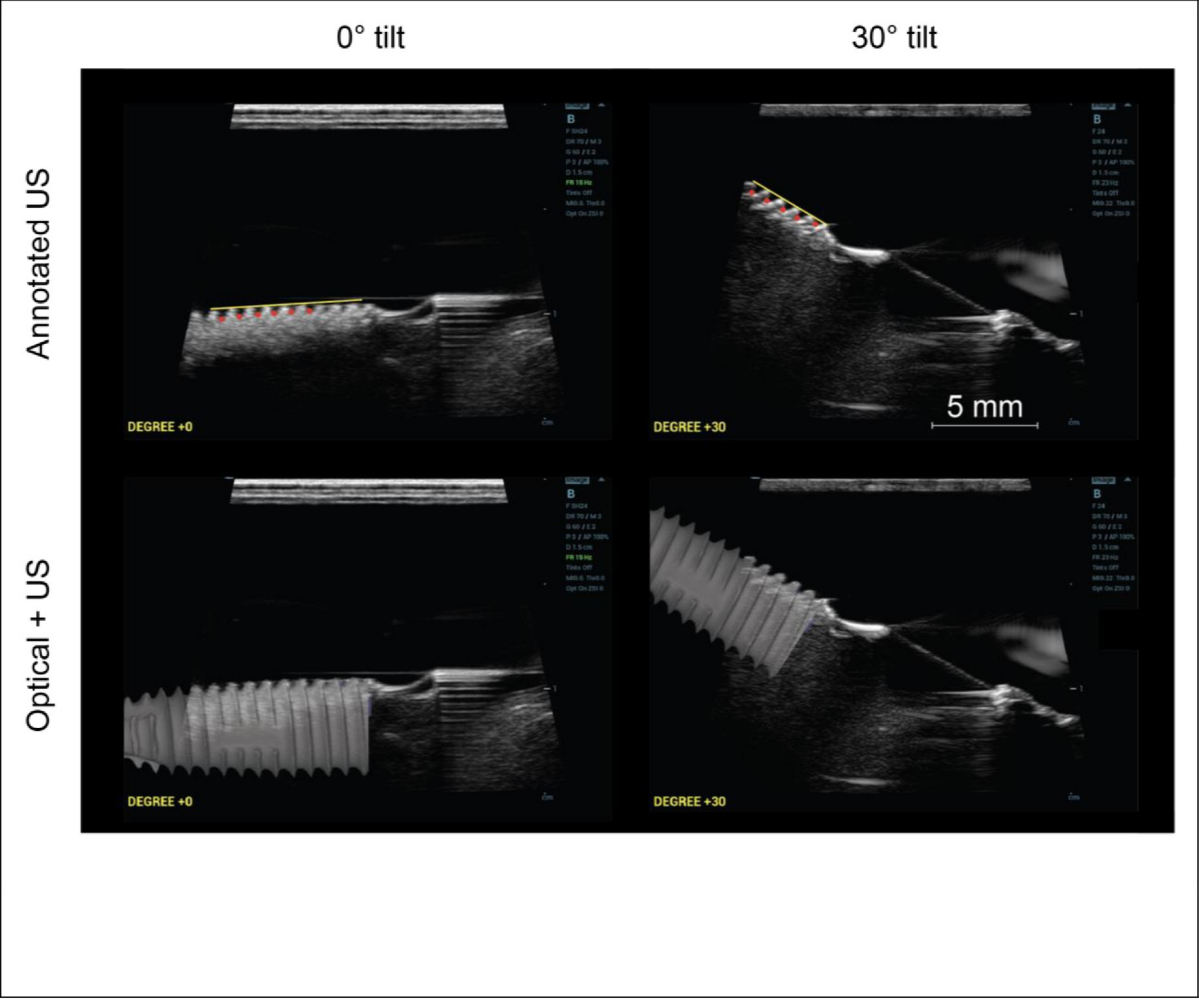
Implant thread pitch and thread depth measurement dependence on the underlying image angle, i.e., angle between the implant and ultrasound array, e.g., DEGREE +0 or +30 in the shown images. Top: A line connecting the peaks of at least 5 consecutive threads is drawn (yellow line). Associated valleys are identified and shown as red dots. The thread depth was measured from the yellow line to each individual red dot and averaged. The thread pitch was measured as the distance between the adjacent red dots that were projected to the yellow line and averaged. Bottom row: Overlaid semi-transparent optical scan of the imaged implant, i.e., a 4.5–13 as shown in S2 Fig. The scale bar is for all images.
